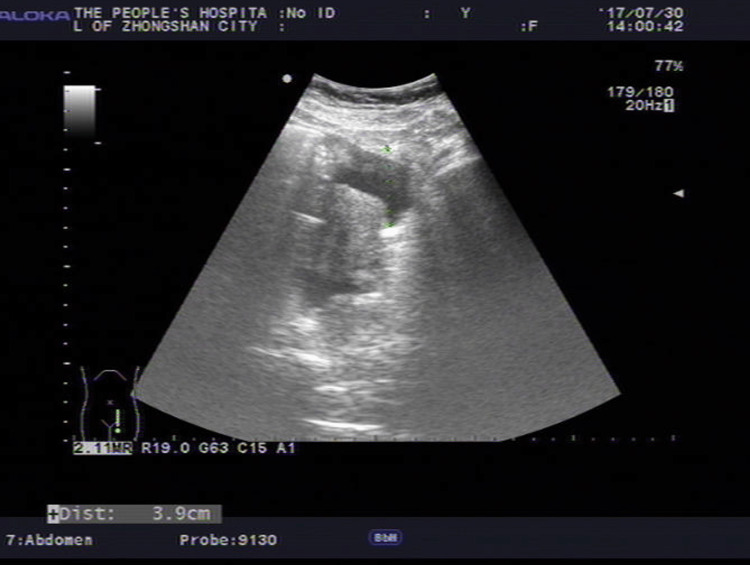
Abdominal ultrasound image of ascites. Ultrasound examination revealed no obvious effusion in the abdomen but a 39 mm fluid sonolucent area in the Douglas pouch.
